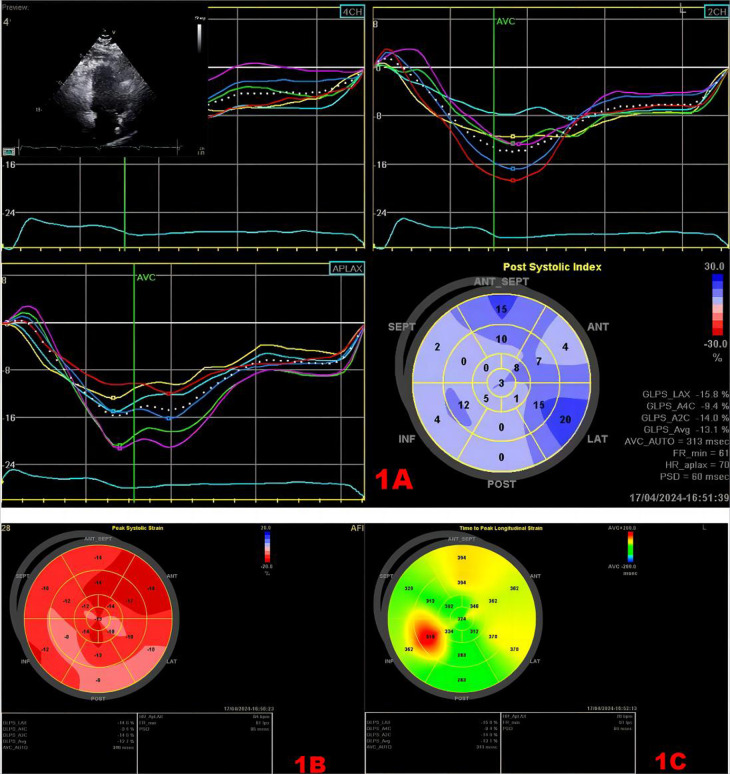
Speckle tracking map. (A) Strain time curve and strain peak time plot. Upper left myocardial Doppler 2D measurement image of the region of interest. The middle curve is the strain–time curve. The lower right panel shows the time-to-peak bull's eye plot, and the values in the lower right panel show the strain values, GLS, and longitudinal strain time-to-peak dispersion in each section. (B) Bull's eye plot of LV longitudinal strain, with approximately red color representing greater myocardial deformability. (C) Bull's eye plot of the time to peak in the left ventricle, the longer the time to peak, the darker the color.
